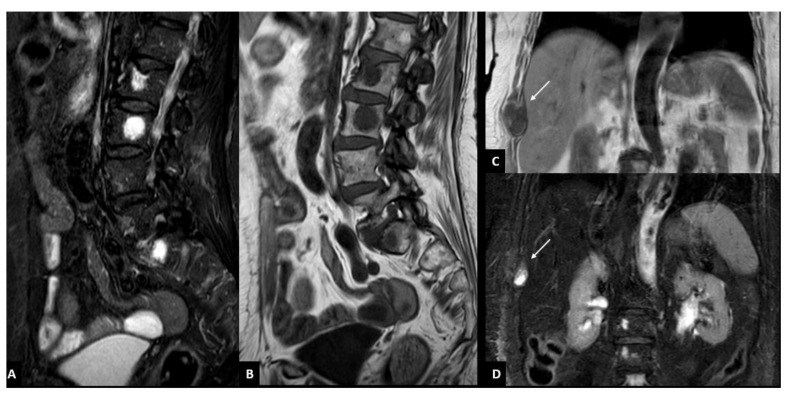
Focal infiltration pattern of multiple myeloma characterized by bone lesions of the spine (A,B) and the rib (C,D). Focal bone lesions of the spine are visible as a focal area of hypointensity on T1-weighted turbo spin-echo sequence (A) and of hyperintensity on T2-weighted short-tau inversion recovery (STIR) sequence (B) in L2, L3, and S1. Focal bone lesion of the rib is visible as focal area (arrow) of hypointensity on T1-weighted turbo spin-echo sequence (C) and of hyperintensity (arrow) on T2-weighted short-tau inversion recovery (STIR) sequence (D) in the costal angle of the ninth right rib.
